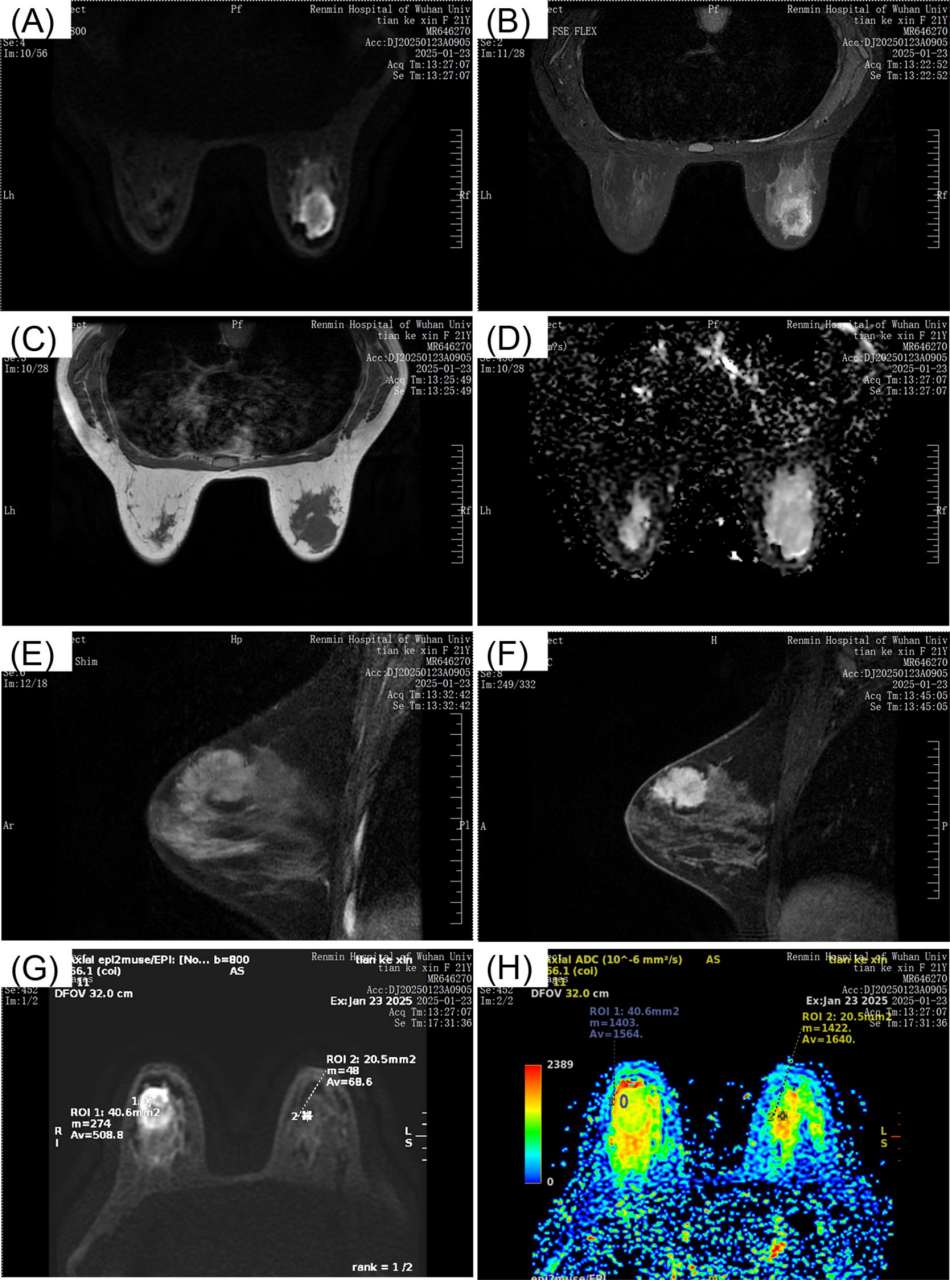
MRI breast scans depicting different views. Image (A) shows a coronal section with a visible mass. Image (B) presents another coronal view. Image (C) is a transverse section. Image (D) displays a diffusion-weighted image. Image (E) and (F) are sagittal views with noticeable lesions. Image (G) and (H) contain color maps indicating apparent diffusion coefficients, with regions of interest marked. All images are labeled with patient information and scan details from Renmin Hospital of Wuhan University, dated January 23, 2025.
